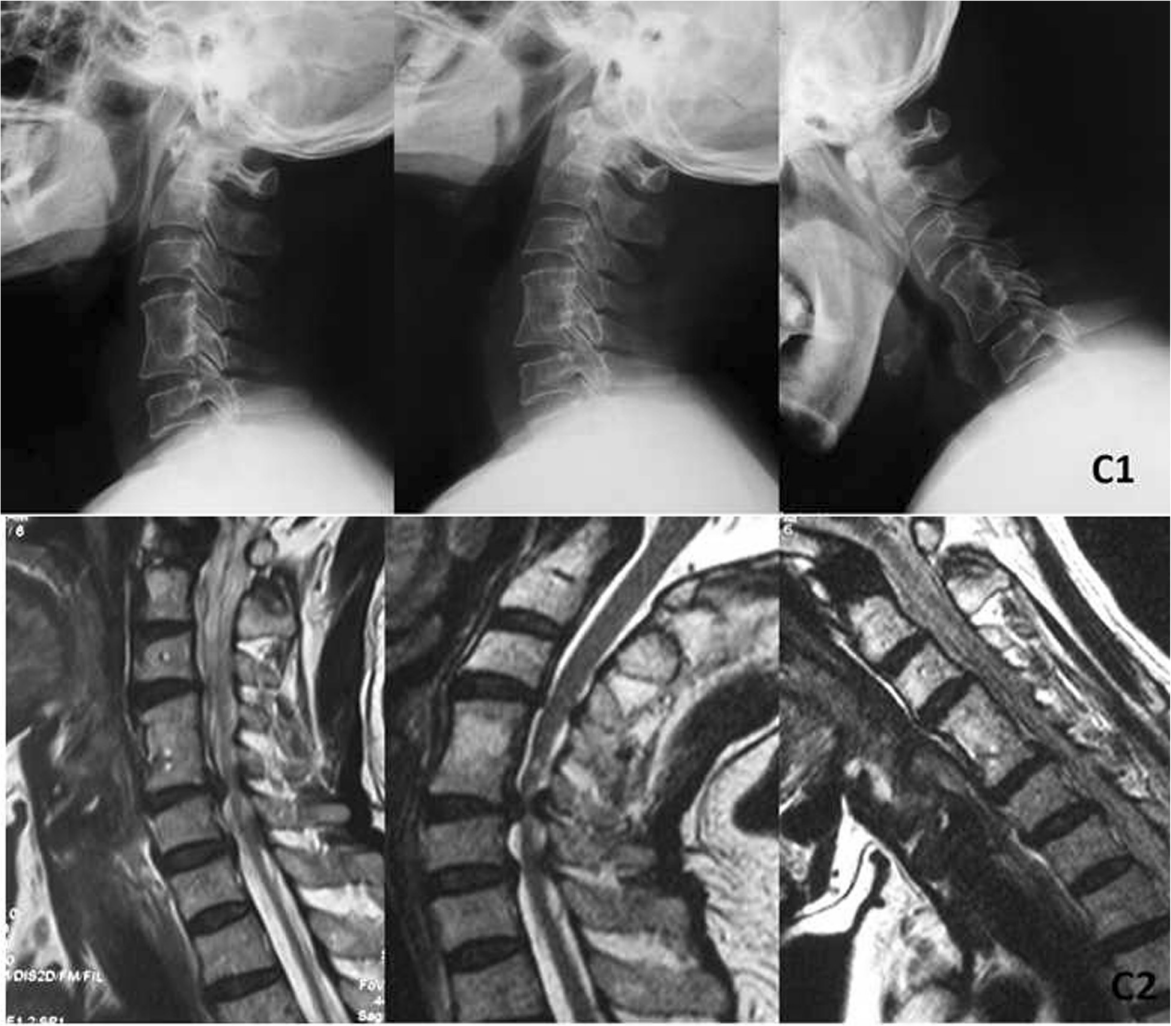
A 58-year-old woman developed one-level adjacent segment disease 12 years after primary surgery. (*C1*) Radiograph and (*C2*) MRI (flexion/extension) at 12 years after operation indicate C5–6 disc hernias
